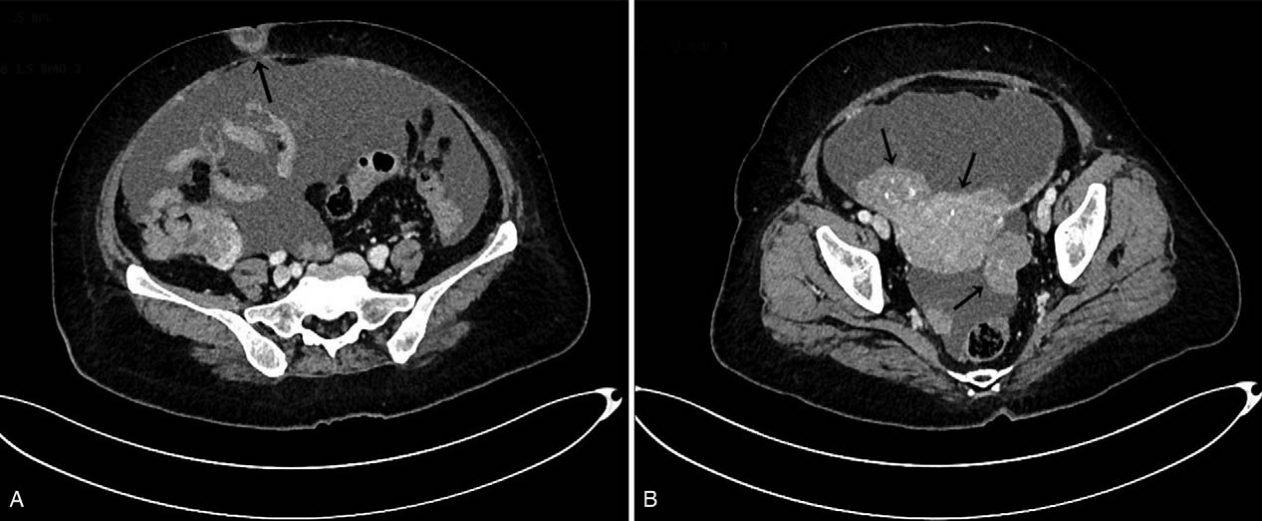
Computerized tomography (CT) scan of the entire abdomen. A nodular soft tissue in the subcutaneous fat space of the umbilical area was observed (A). Furthermore, multiple pelvic masses which were suspected metastases of peritoneal and omentum of ovarian cancer were shown (B).
